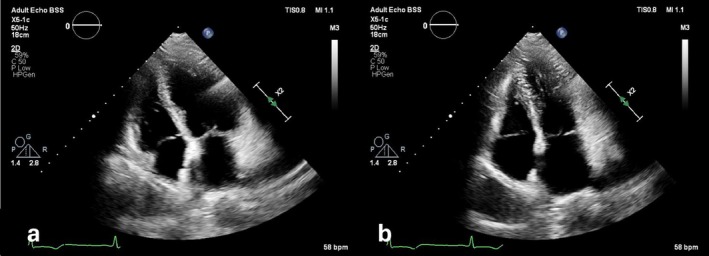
Apical four chamber view on follow up showing apical hypertrophy (a: End diastole; b: End systole).
